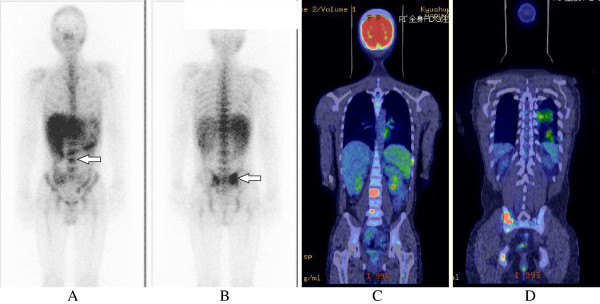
Gallium scintigraphy at hospital day 38 and fluoro-2-deoxy-D-glucose positron emission tomography at hospital day 56. Images of gallium scintigraphy show high up-take (arrows) in the systemic bone marrow (1A) and right sacroiliac joint (1B). Images of fluoro-2-deoxy-D-glucose positron emission tomography (1C and 1D) show abnormal uptake in some ribs, the spine, sacroiliac joint, mediastinal lymph nodes, and bilateral hilum of lung.
